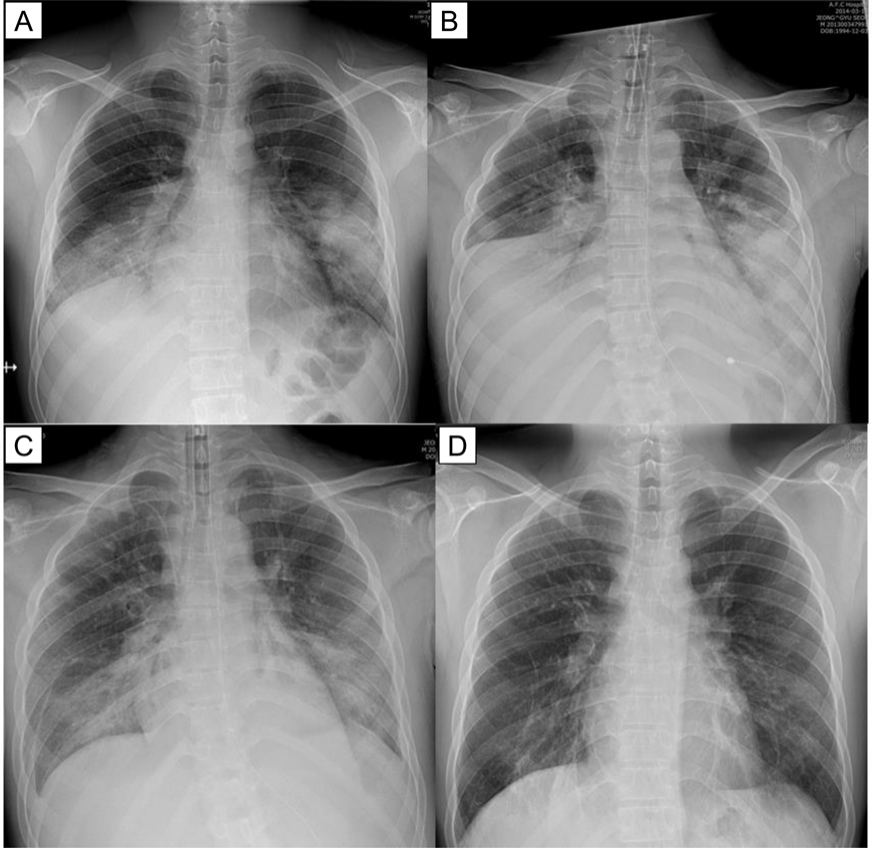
Radiological findings in *patient 1* with severe AdV pneumonia. Chest radiography on admission shows (A) bilateral consolidation in both lower lobes. Next day, the patient was rapidly deteriorated and needed vasopressor and mechanical ventilation (B). Approximately 4 days after cidofovir administration, radiographic resolution occurred (C). Chest radiography obtained after 3 weeks of cidofovir administration shows (D) nearly complete resolution of the previously abnormal radiographic findings.
